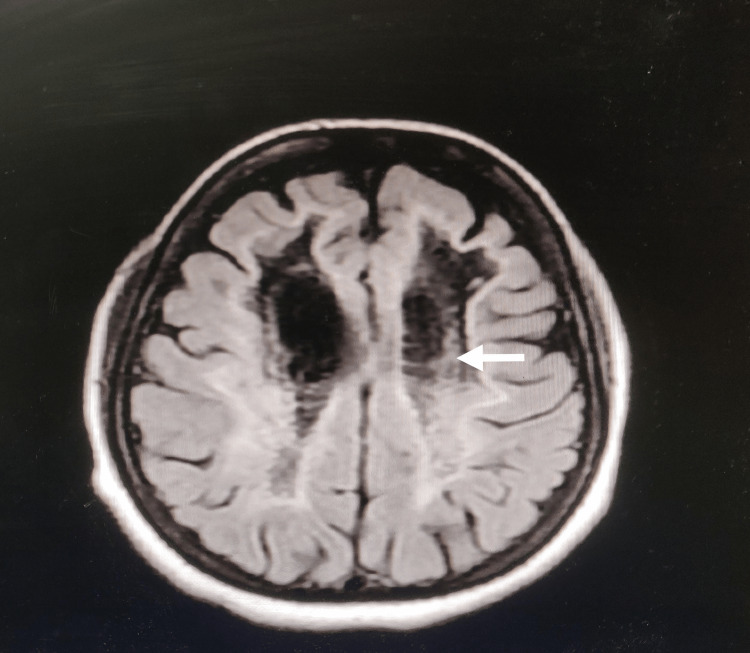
Axial FLAIR image showing cystic degeneration and rarefaction of white matter. FLAIR: Fluid-attenuated inversion recovery
